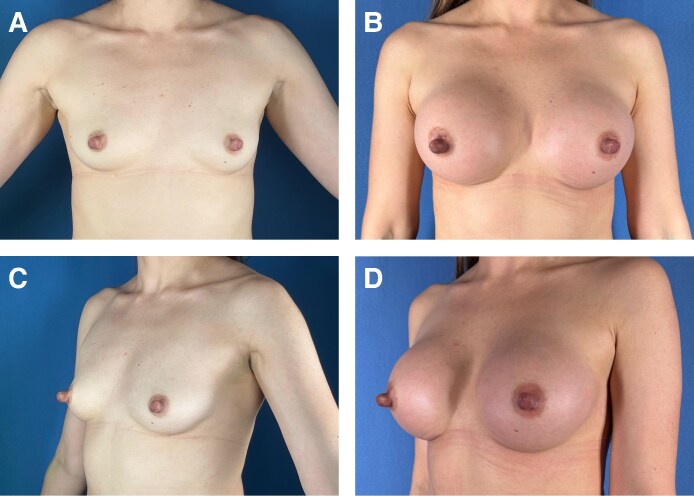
(A, C) Before and (B, D) 6 months after images of a 37-year-old female treated with ultrasonic breast augmentation using 315 cc Motiva Full ergonomix implants.
